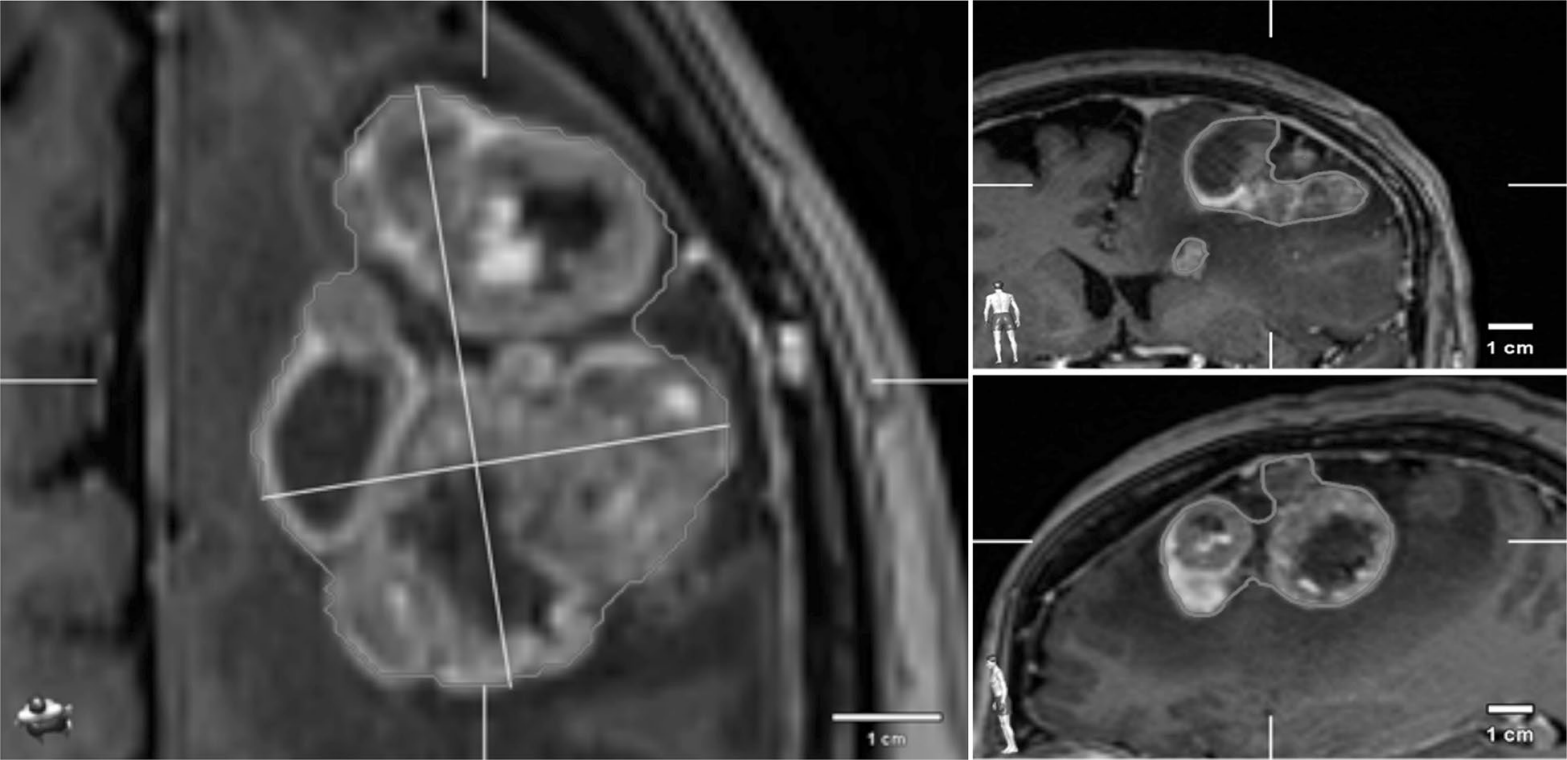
It shows an MRI scan of brain and solid tumor. Contrast was used during MRI examination
